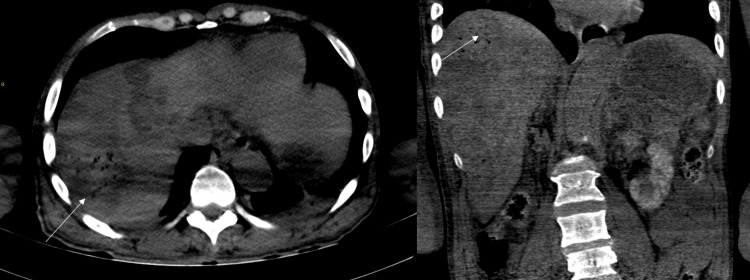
Noncontrast axial and coronal CT scan images. Numerous hypoattenuating lesions throughout the liver with new foci of gas (arrow) can be seen in the right hepatic lobe after TACE in the presence of leukocytosis, and pain is suggestive of post-embolization syndrome. TACE, transarterial chemoembolization
